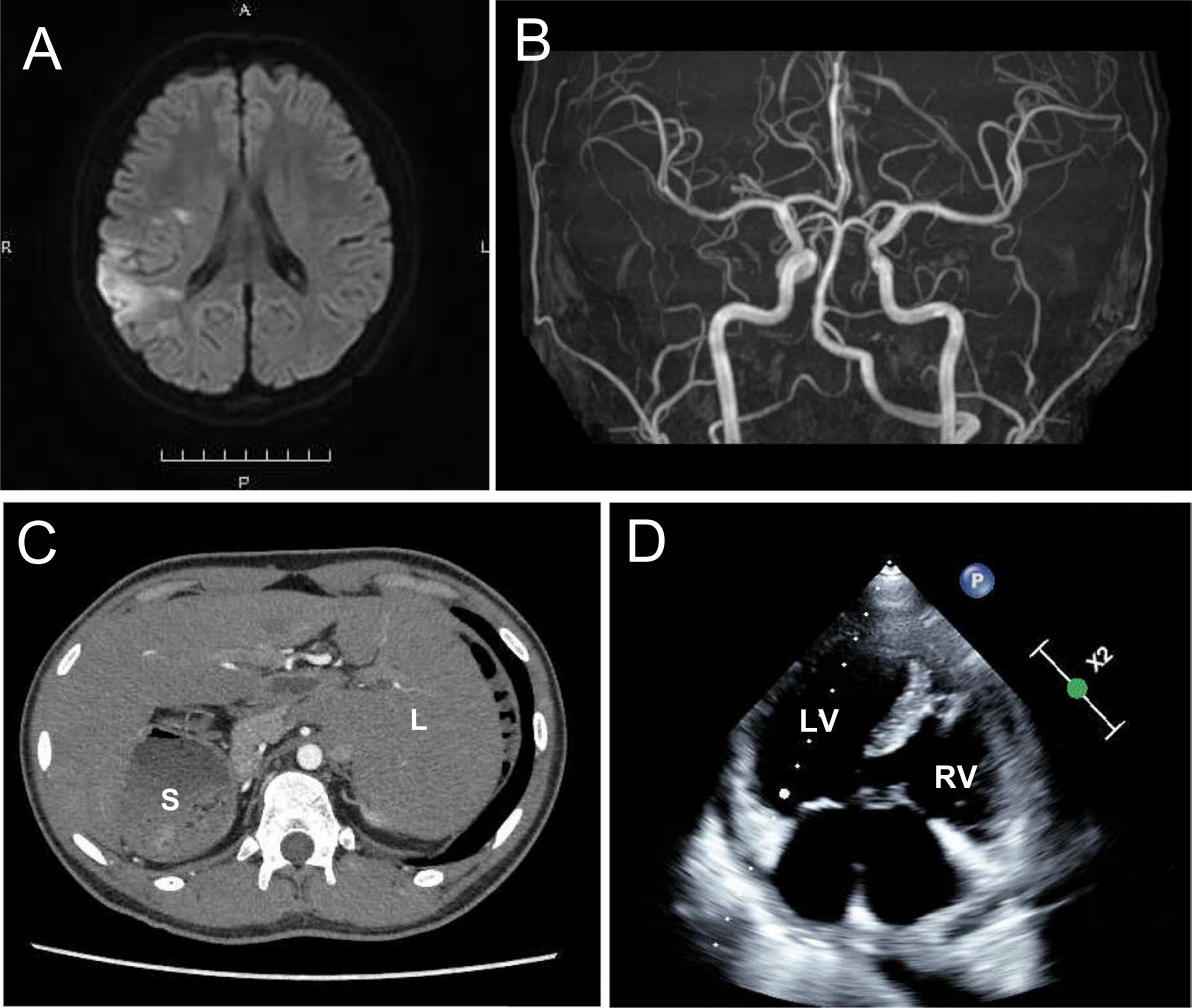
Clinical and radiological features of the patient. A The head MRI (Diffusion weighted image) shows acute cerebral infarction on temporo-parietal lobe. B MR angiography image shows no vessel abnormality. C Abdominal CT shows visceral heterotaxy. S: Stomach. L: Liver. D Echocardiogram. Echocardiogram shows ECD. RV: Right ventricle. LV: Left ventricle
